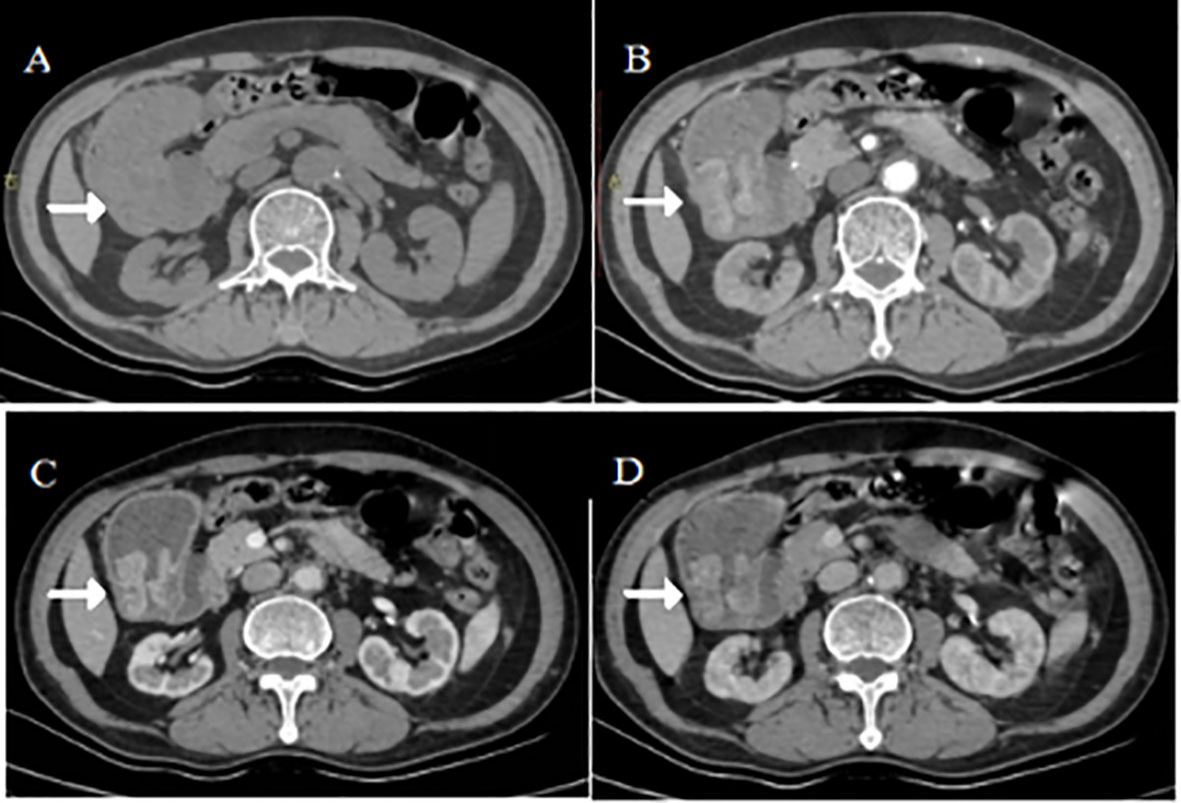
Initial staging CT (February 2020). Axial contrast-enhanced CT images in (A) arterial, (B) portal venous, and (C) delayed phases, with (D) coronal reformat, demonstrate an irregularly thickened, hyperenhancing gastric antral wall (white arrows), characteristic of the primary hepatoid adenocarcinoma.
